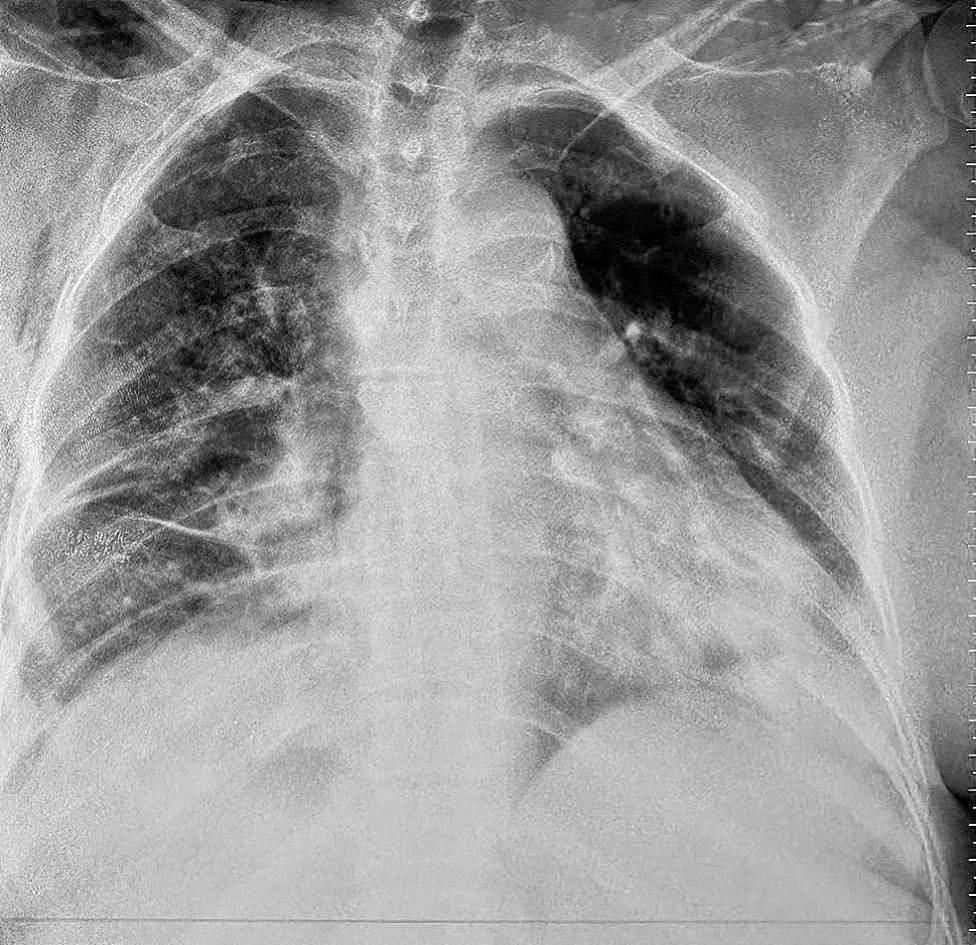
Postoperative Chest Xray showing the disappearance of the opacity
